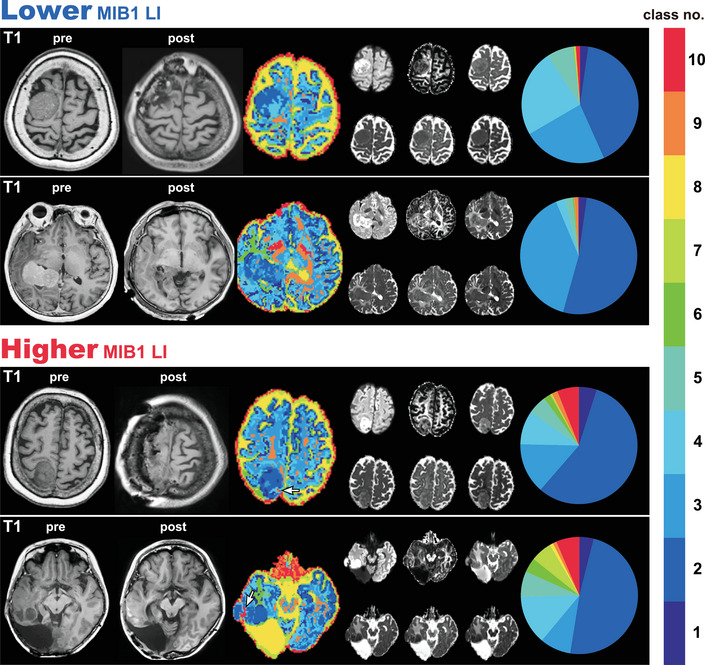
Representative cases of meningiomas with lower and higher MIB‐1 LIs, including the 10‐class DTcIs that showed the highest classification performance. The preoperative and postoperative T1‐weighted images, DTcIs, six diffusion tensor images, and the ratios in each class number are shown for each patient. The arrows show the areas of warm‐colored classes, which indicate the areas with higher MIB‐1 LI. The colors on the DTcIs and circular charts correspond to the class numbers, which are shown in the color bar. MIB‐1 LI, Ki‐67 monoclonal antibody labeling index; DWI, diffusion‐weighted imaging; FA, fractional anisotropy; S0, raw T2 signal without diffusion weighting; MD, mean diffusivity; AD, axial diffusivity; RD, radial diffusivity; DTcI, diffusion tensor‐based clustering image.
